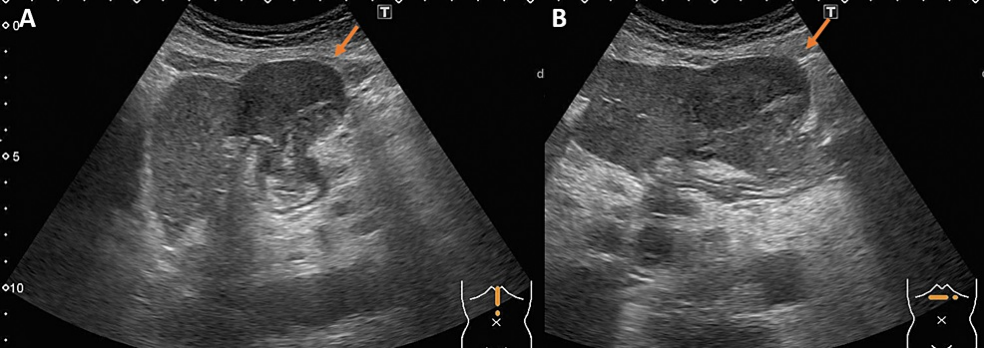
Abdominal ultrasonography (A) longitudinal axis, (B) horizontal axis A 4.5-cm, internally heterogeneous, hypoechoic mass is contiguous from the stomach to the liver.
